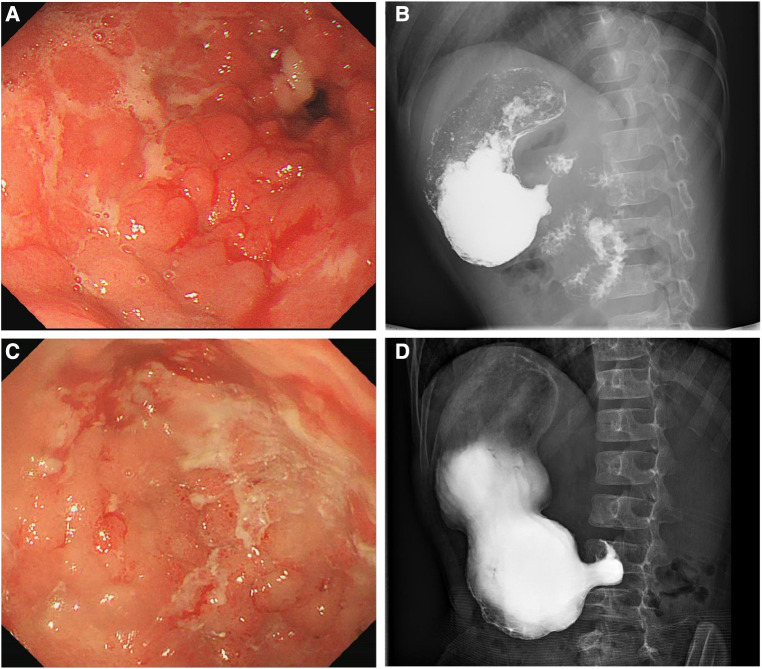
Esophagogastroduodenoscopy (EGD) showing edema, erosion, multiple ulcers, and pyloric stenosis of the stomach at 7 (A) and 12 (C) years old. Upper gastrointestinal radiography showing gastric retention and pyloric stenosis at 7 (B) and 12 (D) years old.
